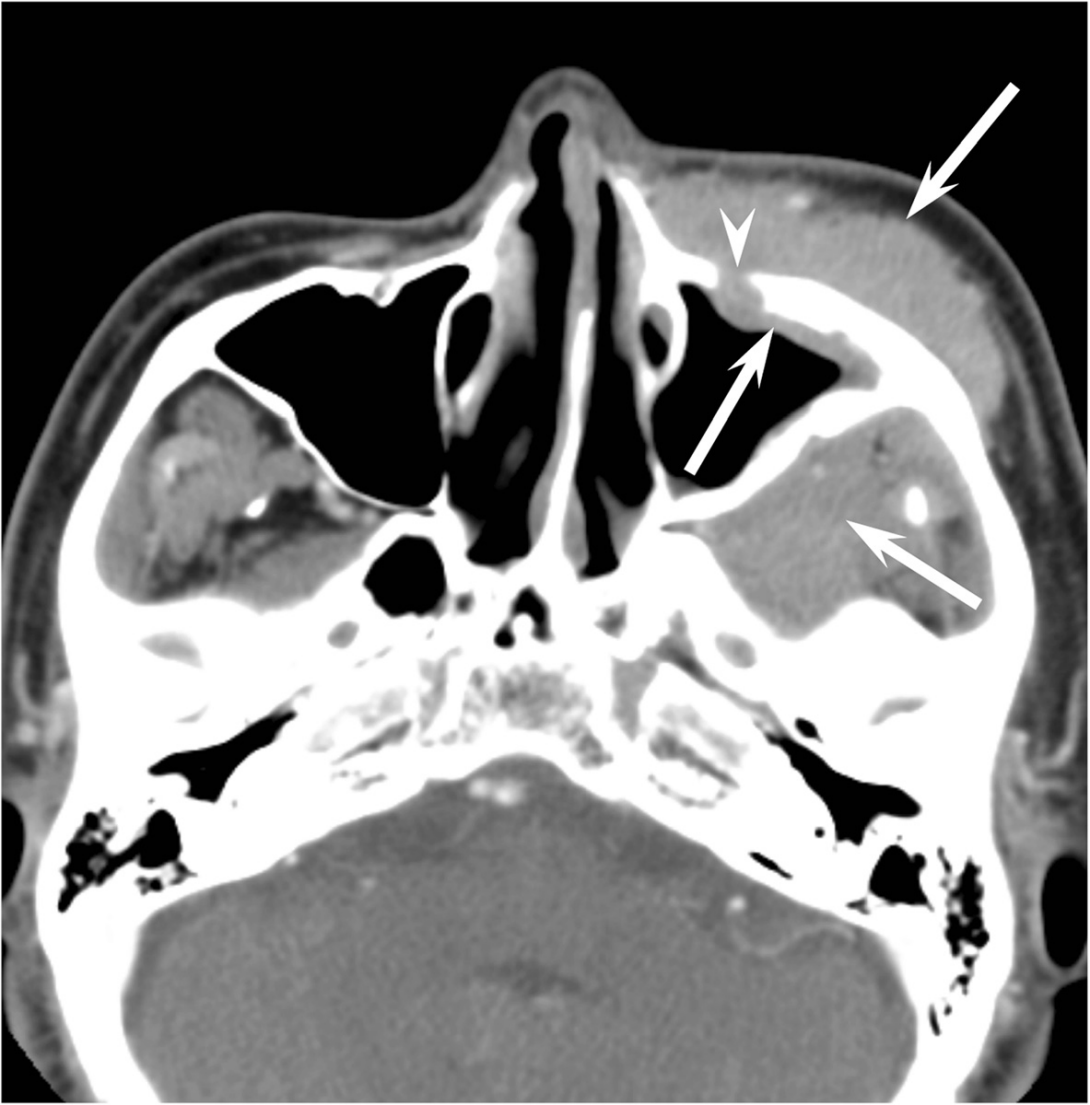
A 50–year-old man with maxillary sinus diffuse large cell lymphoma (permeative-type). Enhanced CT image shows a homogeneously enhanced mass inside and outside left maxillary sinus (arrow). The sinus walls are preserved due to the permeative growth, but infraorbital foramen (arrowhead) is slightly expanding
